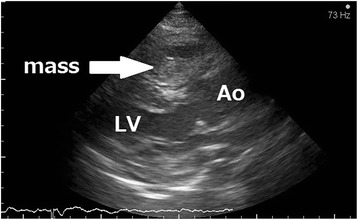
Echocardiography showing the right ventricle compressed by a mass. LV, left ventricle ; Ao, aorta
